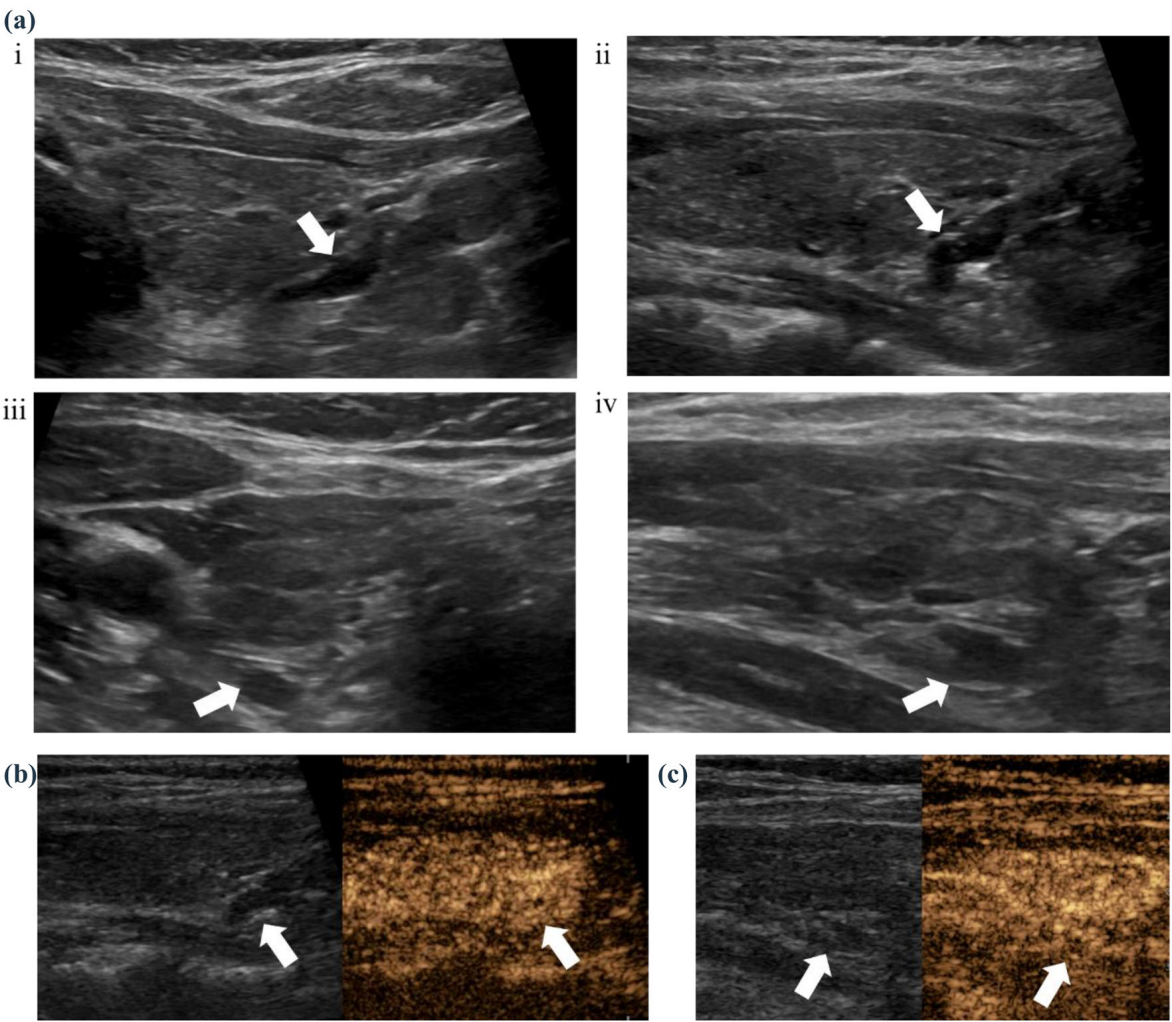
A 51‐year‐old‐female patient with HPHT. Pathology showed that the left lower pole lesion was lymph node and the right lower pole lesion was parathyroid adenoma. (a) Ultrasonography (US) shows a hypoechoic nodule behind each of the right thyroid lobe ((i) cross section, (ii) longitudinal section, white arrows) and the left thyroid lobe ((iii) cross section, (iv) longitudinal section, white arrows), considering parathyroid adenoma; (b) the hypoechoic nodule at the right lower pole showed overall high enhancement in arterial phase (white arrows), considering parathyroid adenoma, and (c) contrast‐enhanced ultrasound (CEUS) showed that the lesions in the arterial phase of the left lower very hypoechoic nodule showed enhancement of hilus like structure (white arrows), considering lymph nodes.
